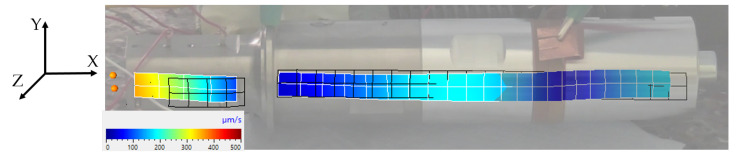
Longitudinal vibration shape of the ultrasonic system.
